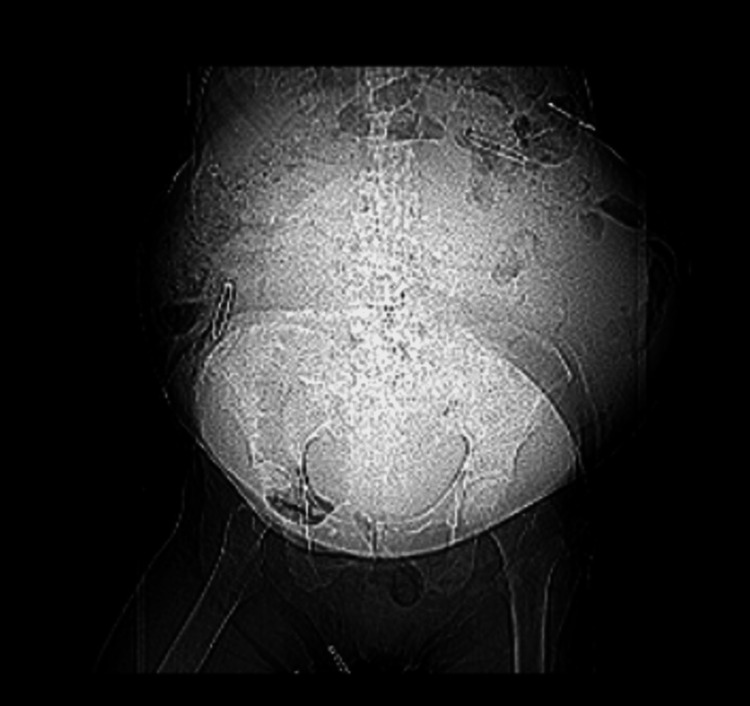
A coronal abdominal CT scan without IV contrast shows the 33.5 cm mass causing significant abdominal distention, correlating with the patient's clinical picture. CT: computed tomography, IV: intravenous
